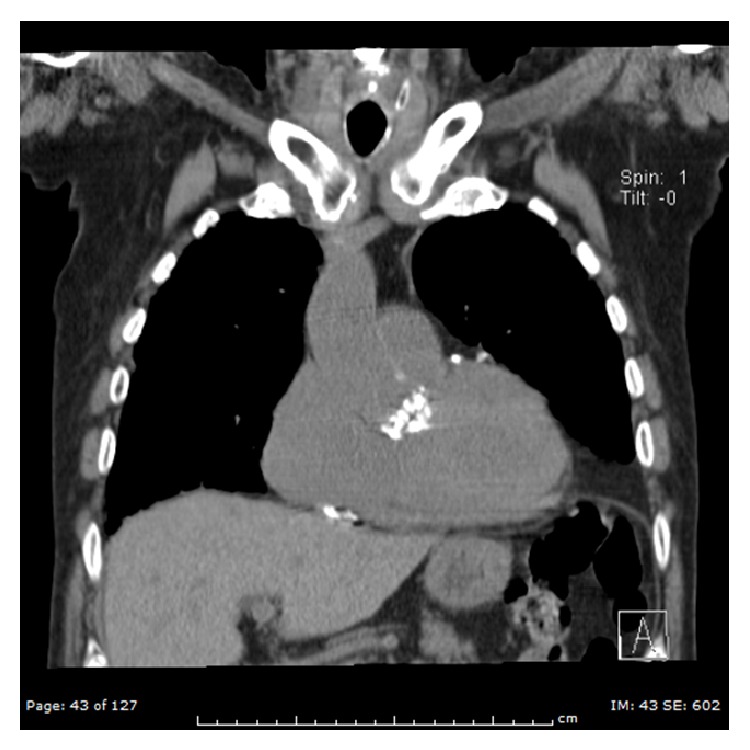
Coronal section of CT chest showing heavily calcified aortic valve.
